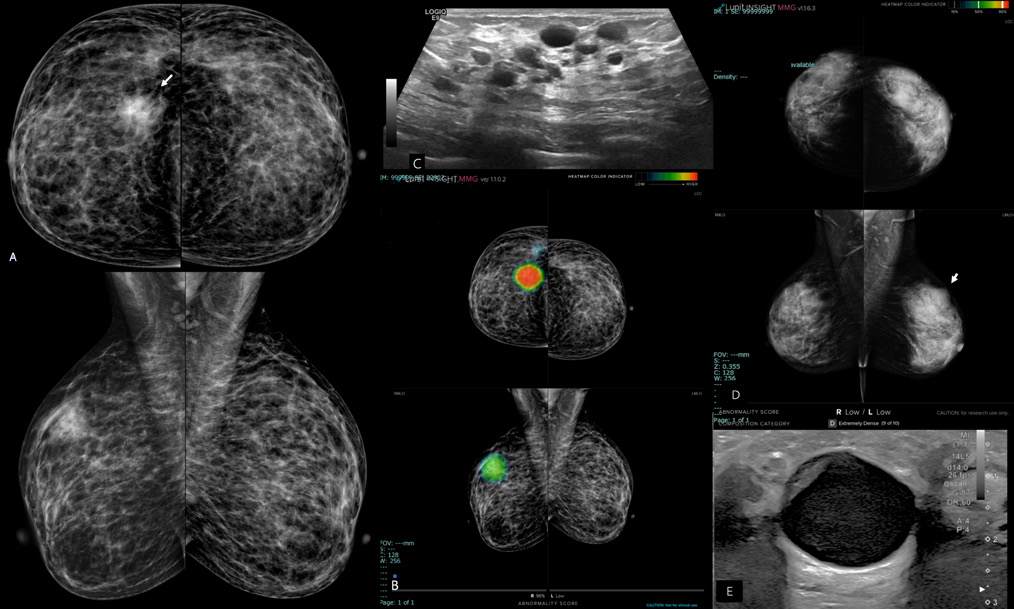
Two cases; 4A, 4B and 4C, right breast atypical ductal hyperplasia in a heterogeneously dense breasts of a 37-year-old female (ACR c). A: bilateral mammograms showed right breast upper outer quadrant indistinct high dense abnormality likely mass (arrow). B, mammograms scanned by the AI algorithm displayed localized intense red color of the detected right breast abnormality and a very high abnormality scoring of 96% suggestive of most likely carcinoma. C, Ultrasound image of the right breast abnormality presented a cluster of small slightly turbid cysts (BI-RADS 3). The case with the aid of ultrasound was a true negative while it was a false positive for AI. Second case; 4D and 4E, female patient 50-year-old with dense breasts (ACR d), that had a left breast upper outer quadrant complicated large cyst. D: Artificial intelligence -aided mammograms displayed “Low” scoring although there is an obvious left breast upper outer quadrant mass of partly circumscribed, partly obscured border (arrow). E, ultrasound image of the left breast showed large cystic mass with turbid echogenic content and mural based non-soft tissue solid component
